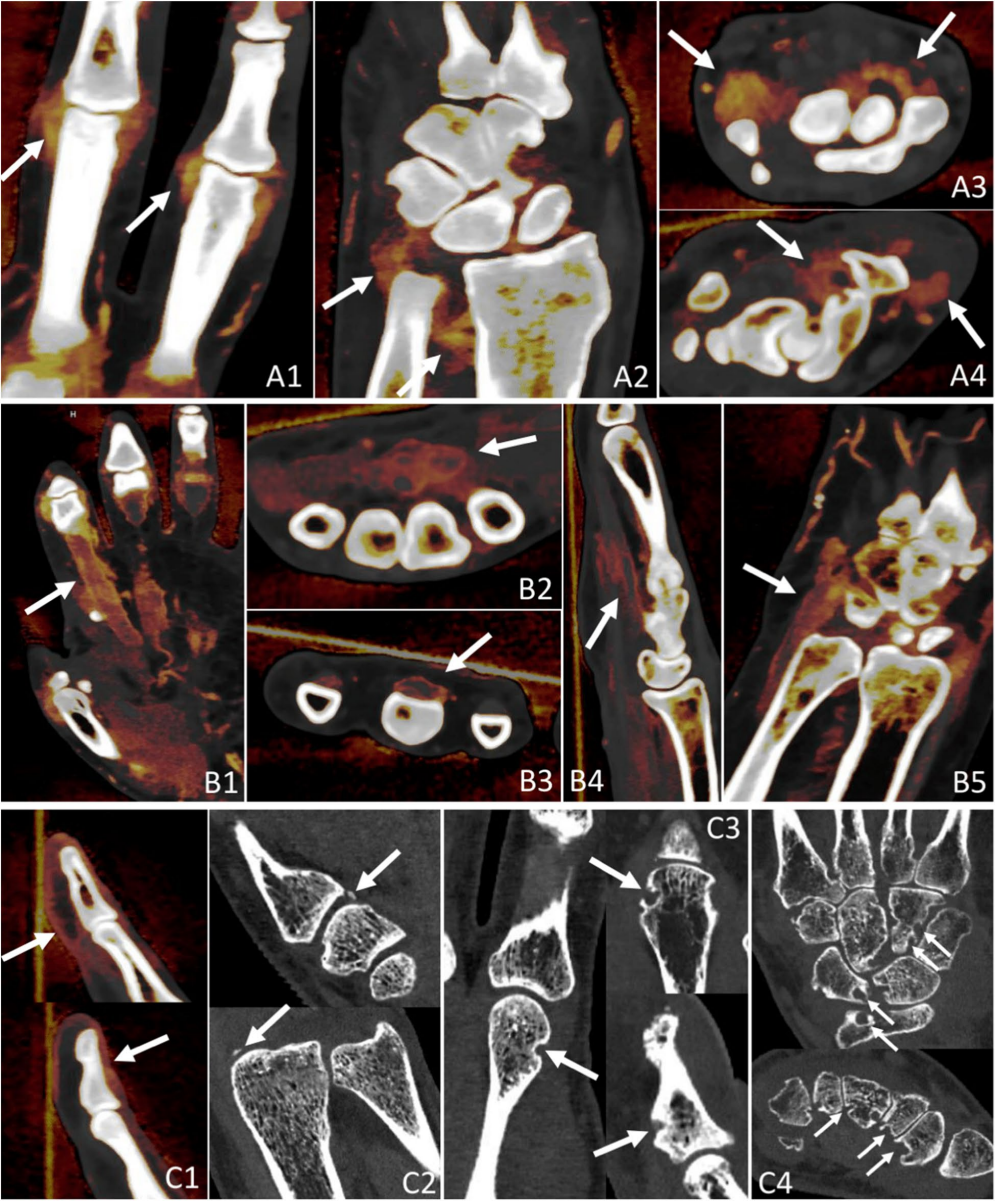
Typical findings in inflammatory peripheral arthritis seen on our PCD-CT images. PCD-CT iodine maps provide high iodine contrast, accurately delineating inflammation, while also displaying bones with high resolution. Synovitis appears as areas of high iodine concentration, represented in red, reflecting thickened and hypervascular synovium, as demonstrated in images A1–A4. This thickening is often nodular due to pannus formation, as seen in A3 (right arrow). Tenosynovitis exhibits similar imaging characteristics to synovitis, with high iodine concentration indicating inflammation, as shown in B1–B5. Periungual soft tissue inflammation, characteristic of psoriatic arthritis, is evident on PCD-CT iodine maps, as demonstrated in C1. In this particular case, the inflammation was detected exclusively on PCD-CT, as neither ultrasound nor MRI identified it. Periostitis, appearing as juxtaarticular new bone formation, also characteristic of psoriatic arthritis, is visible on PCD-CT images (C2), and this finding was also uniquely detected with PCD-CT. Cortical bony erosions of varying shapes and sizes, indicative of longstanding arthritis, are well demonstrated in C3 and C4. CT remains the most sensitive imaging modality for detecting erosions, which serve as a critical marker of disease progression. MRI, magnetic resonance imaging; PCD-CT, photon-counting detector CT
